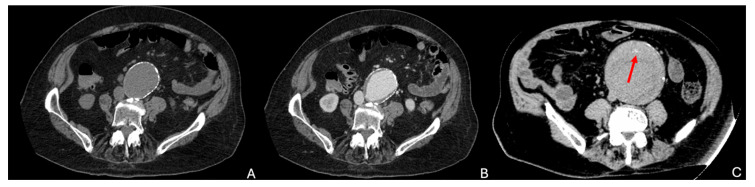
Signs of impending rupture. (A) Unenhanced CTA showing focal discontinuity of calcifications in a large infrarenal abdominal aneurysm (6.7 cm) in a patient with symptoms of abdominal pain. (B) Enhanced CTA showing the low thrombus-to-lumen ratio in the same patient. (C) Unenhanced CTA showing the hyper-attenuating crescent sign (red arrow). CTA: computed tomography angiography.
